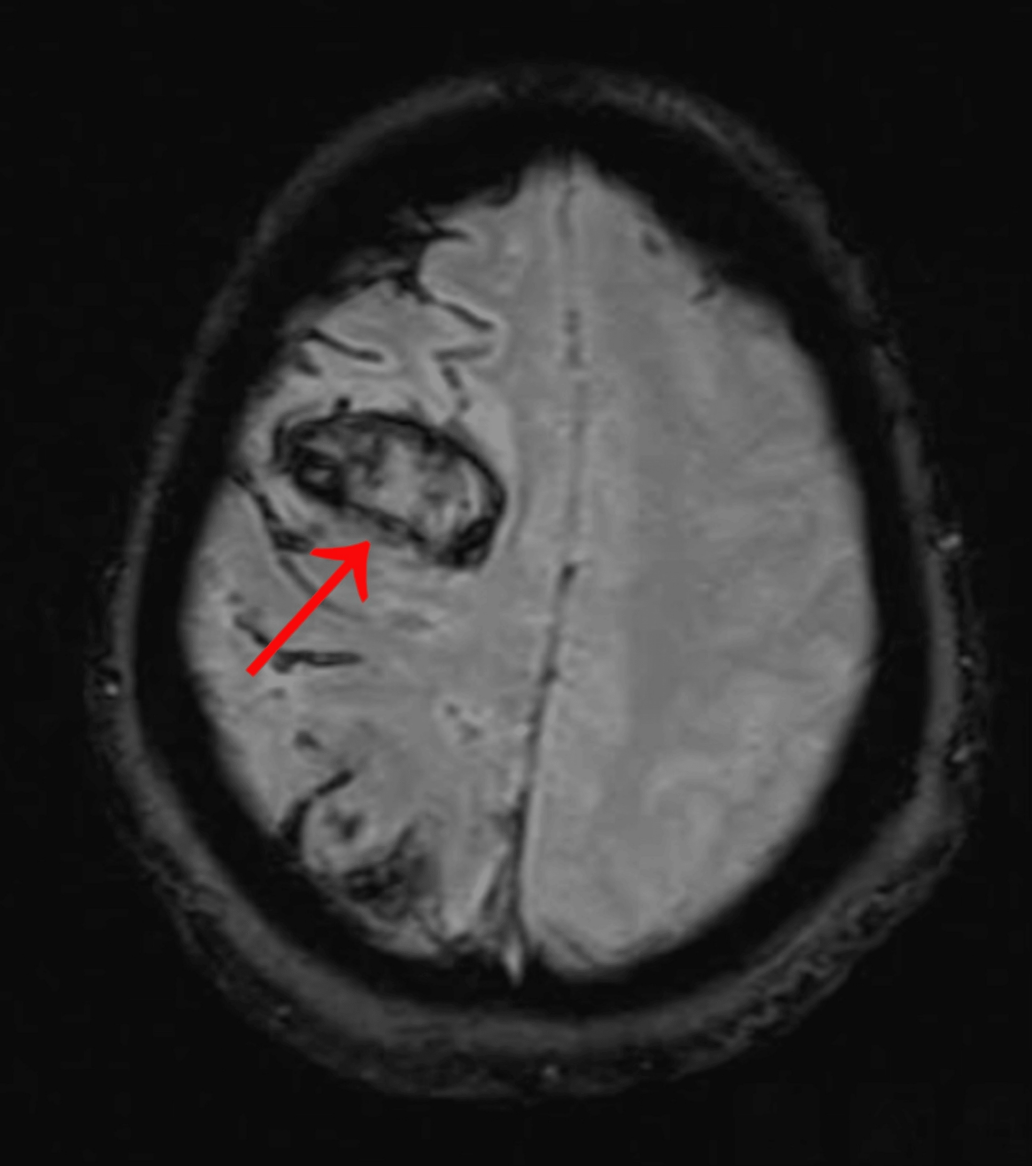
A Gradient Echo (GRE) MRI from August 2022 reveals a large right middle cerebral artery territory infarction, as indicated by the arrow.
